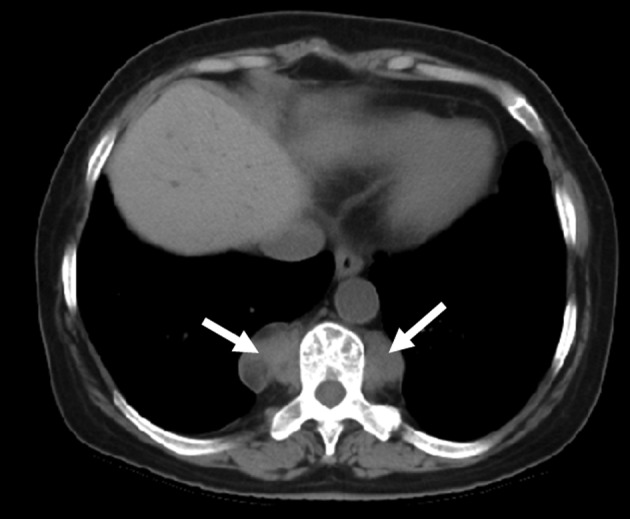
Chest computed tomography (CT) scan reveals two well-defined posterior mediastinal masses containing patchy areas of necrosis (arrows).
